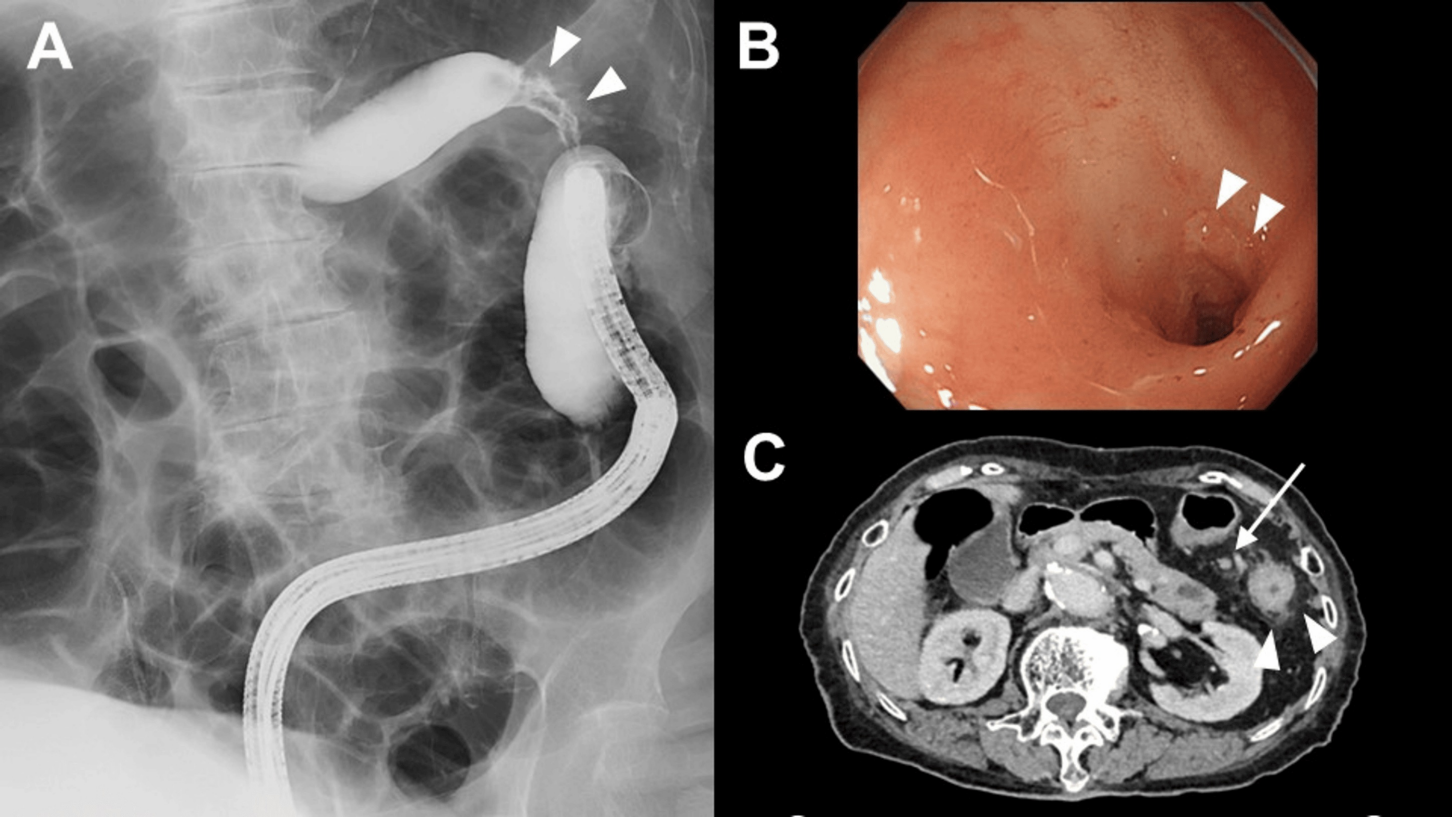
Preoperative findings. (A) Lower gastrointestinal imaging. A circumferential stenosis was observed in the descending colon around the splenic flexure (arrowheads). The length of the stricture was approximately 3.5 cm. (B) Colonoscopy finding. A circumferential stenosis of the descending colon was observed. A longitudinal ulcer was observed in the center of the stenosis (arrowheads). Colonoscopy could not pass through the stenosis. (C) Enhanced abdominopelvic CT. CT scan showed the thickened descending colon wall (arrowheads) and the swollen lymph nodes around the descending colon (arrow) CT: computed tomography
